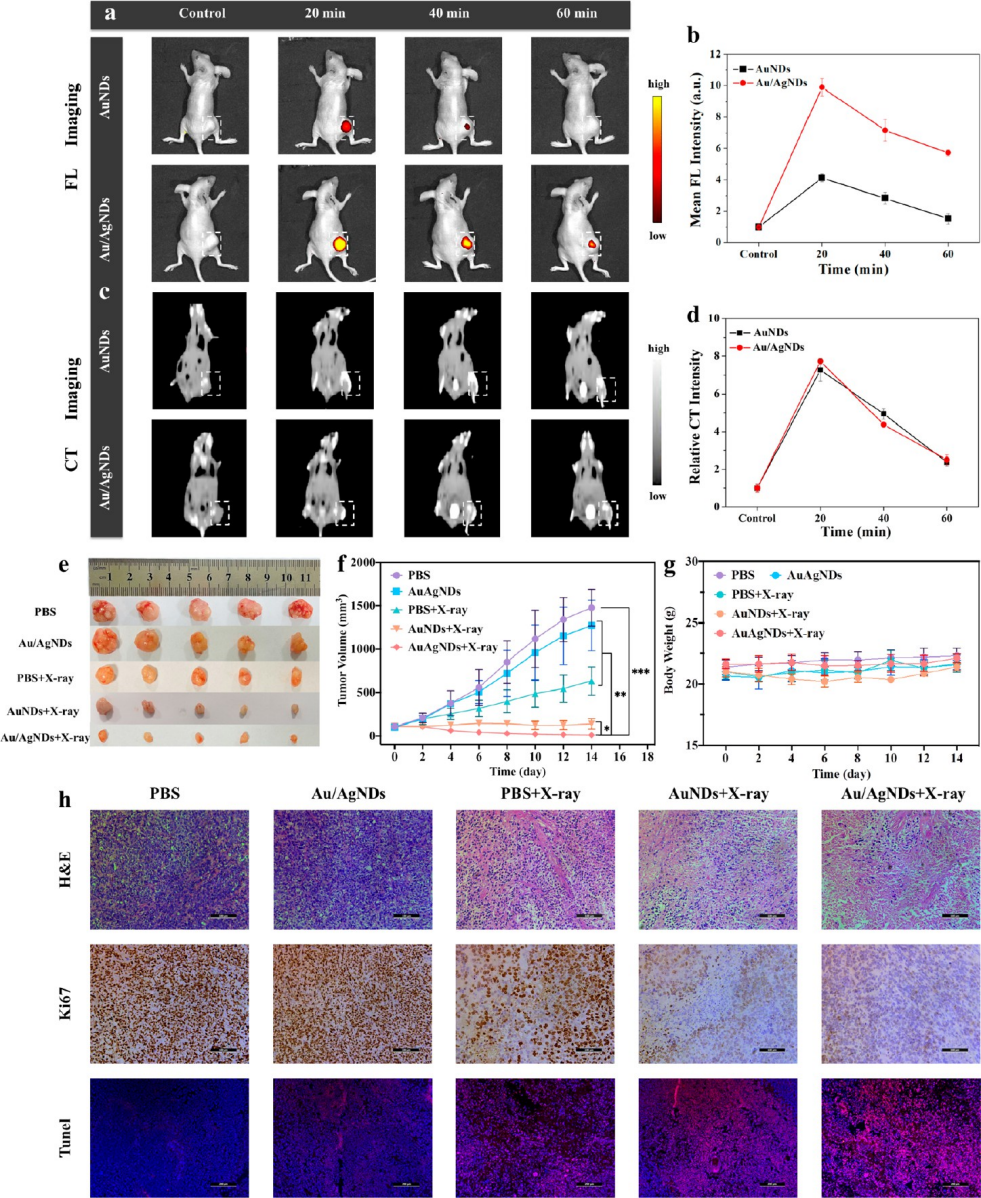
FL/CT dual-mode imaging-guided RT in vivo. (a) FL and (c) CT images
